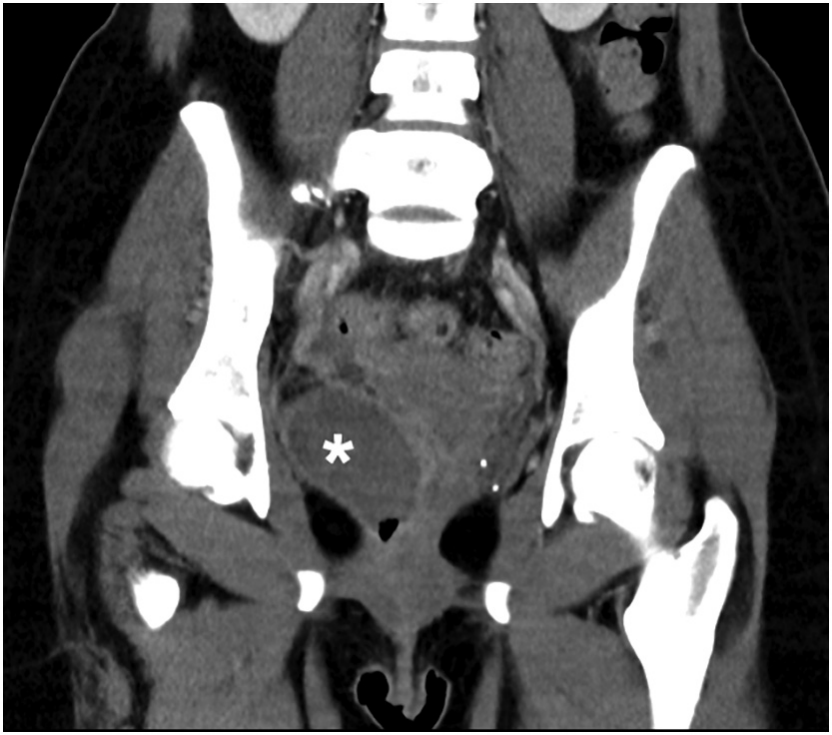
CT scan 2 weeks postpartum, demonstrating reduced paravaginal haematoma*.
